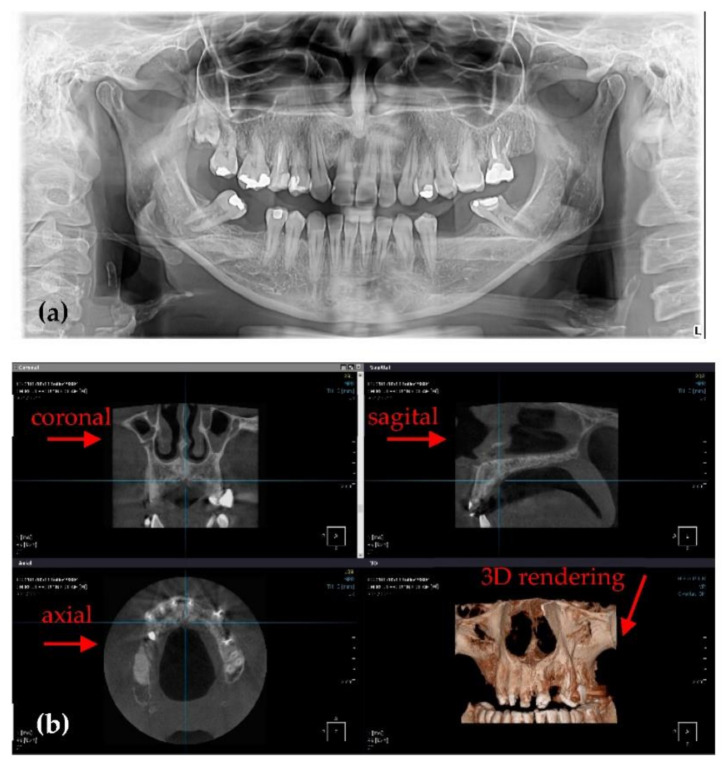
Examples of images obtained with Soredex setups (for a healthy member of the clinic’s staff): (a) panoramic and (b) 3D CBCT, the latter showing coronal, sagittal, axial views, as well as a 3D volumetric reconstruction.
